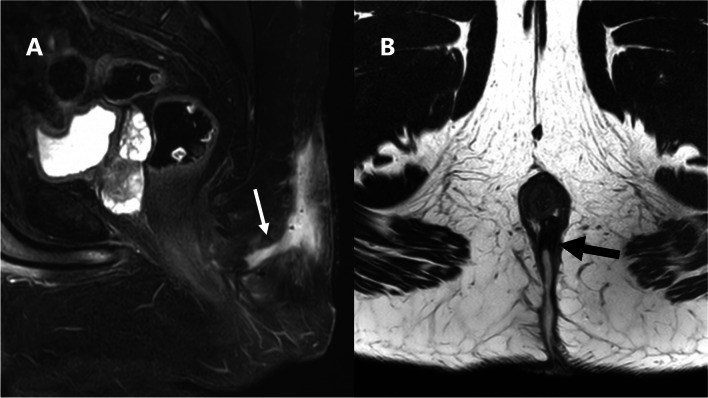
Sagittal STIR-weighted image (A) and axial T2-weighted image (B) of a 25-year-old patient complaining of recurrent natal cleft discharge after repeated operative interventions. A showing a large retro-coccygeal fluid collection with a caudal branch extending anteriorly within the posterior aspect of the perianal region, reaching the sphincteric complex (white arrow). B shows the caudal branch traversing the midline aspect of the deep external sphincter opposite 6 o’clock position to reach the left posterior quadrant of the intersphincteric plane, yet no definite penetration of the internal anal sphincter (black arrow)
